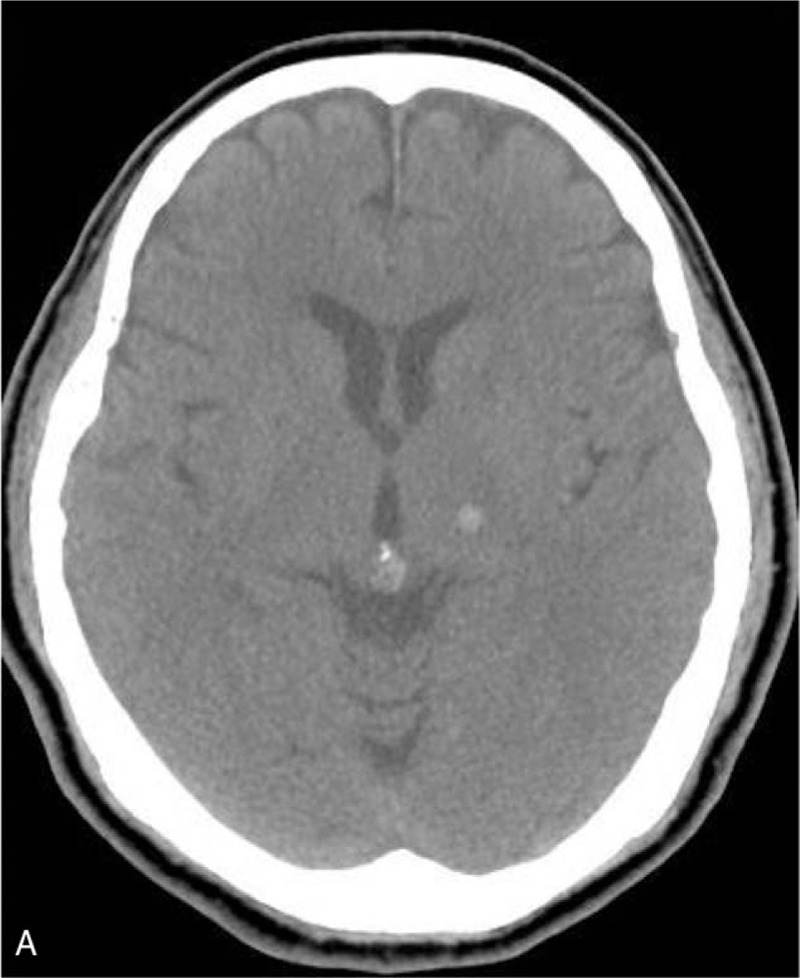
Computed tomography showing left thalamus hemorrhage (axial view).
